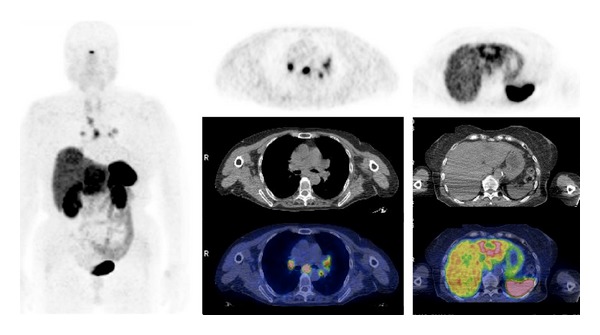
^68^Ga-DOTATOC PET/CT images (MIP, axial) in a patient with metastatic breast cancer.
